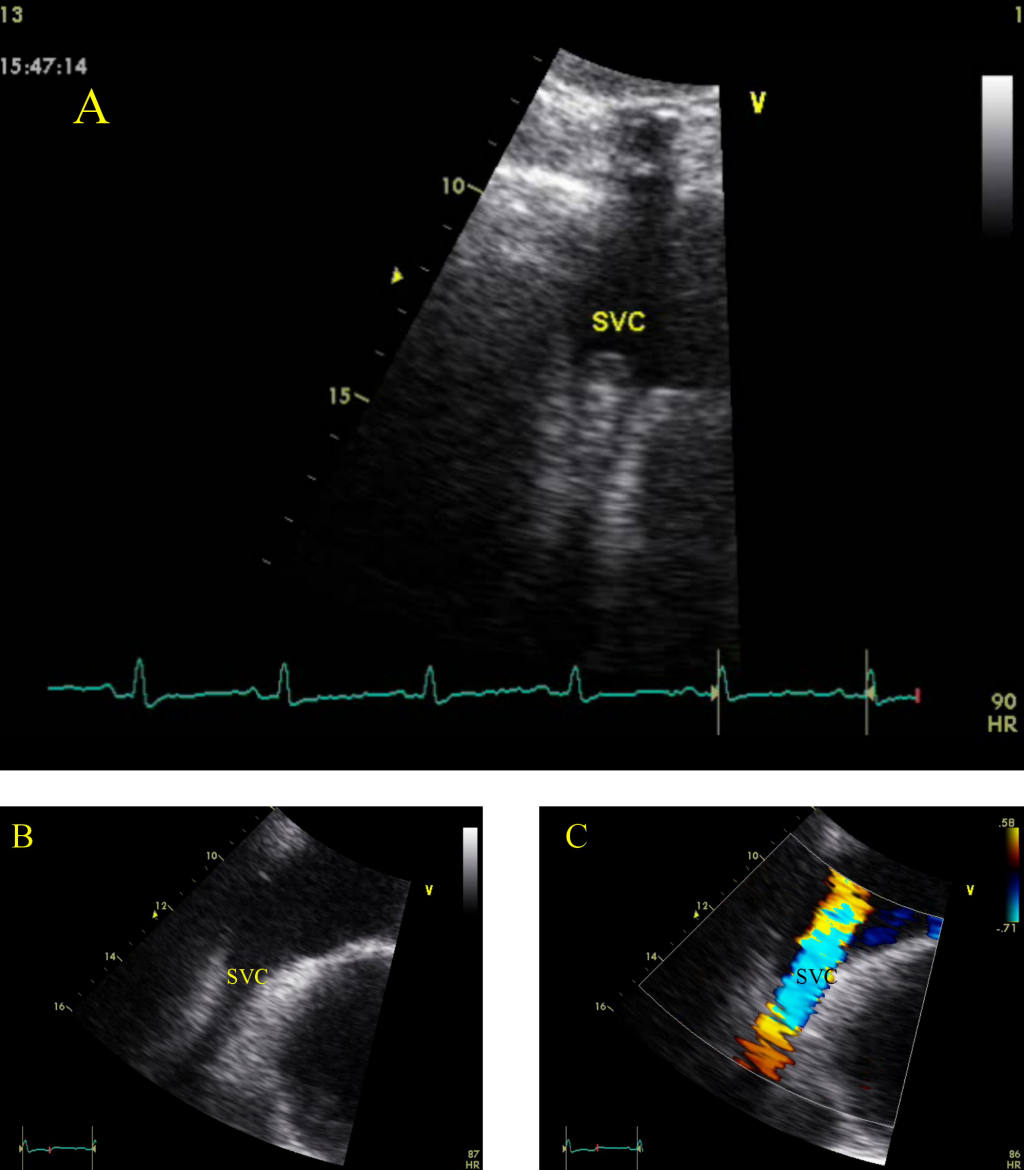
Transthoracic echocardiographic imaging of right atrium and superior vena cava (subcostal view). A round solitary lesion, protruding into the right atrium is clearly visualized (A). The lesion has been diminished 15 days after anti-fungal and anti-thrombotic treatment (B, C).
